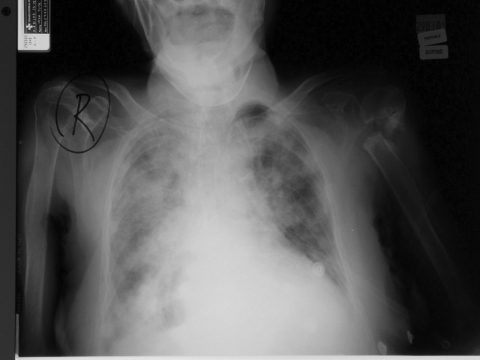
Repeat chest radiograph as second admission.
